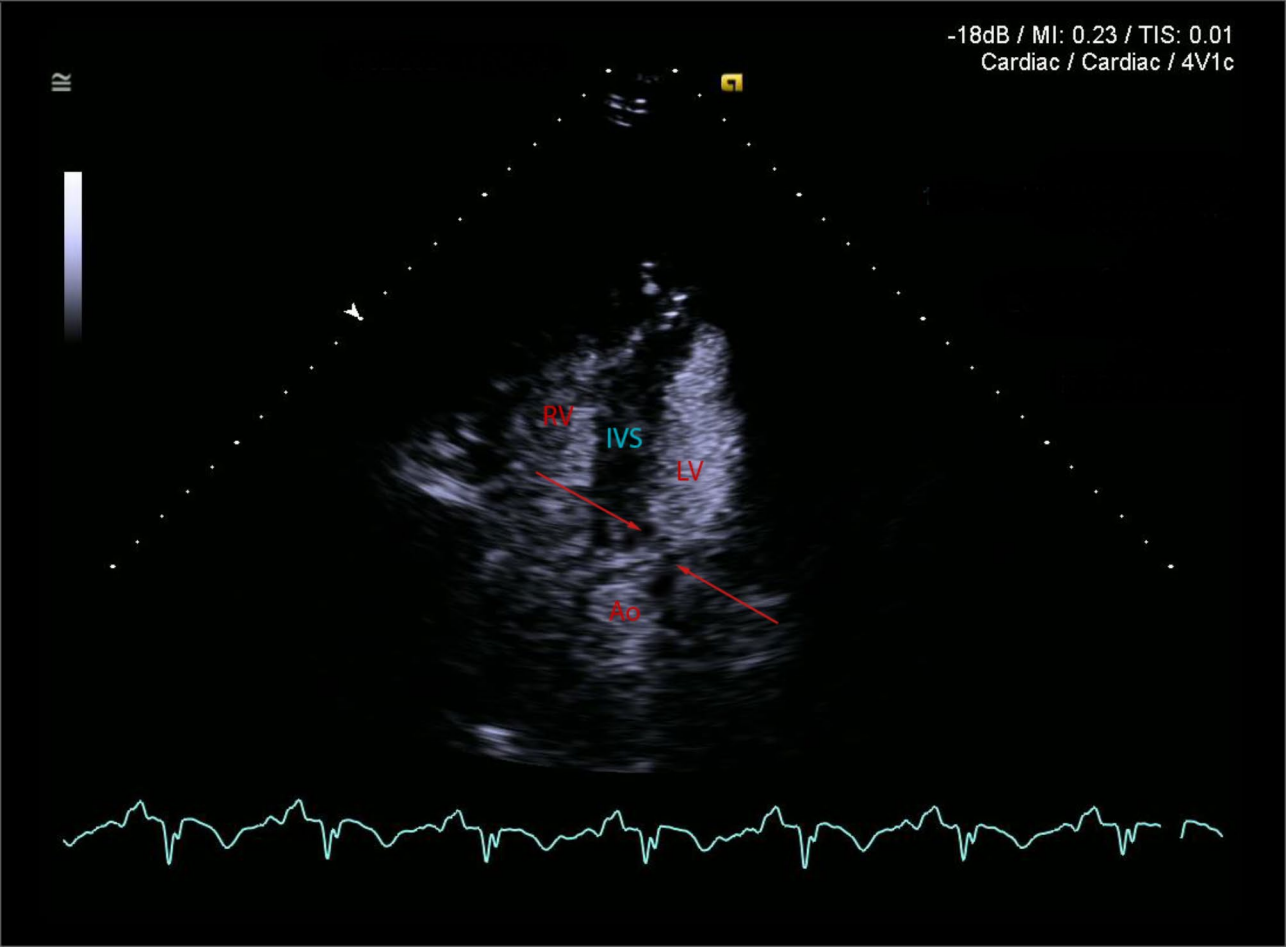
Systolic frame of the apical 5-chamber TTE view with administered ultrasound enhancing agent and low mechanical index settings of the scanner. Narrowing of the LVOT is noted (red arrows)
